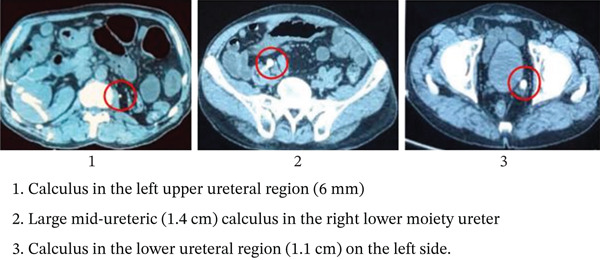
Noncontrast CT KUB. Calculus in the left upper ureteral region (6 mm). Large mid‐ureteric (1.4 cm) calculus in the right lower moiety ureter. Calculus in the lower ureteral region (1.1 cm) on the left side.
